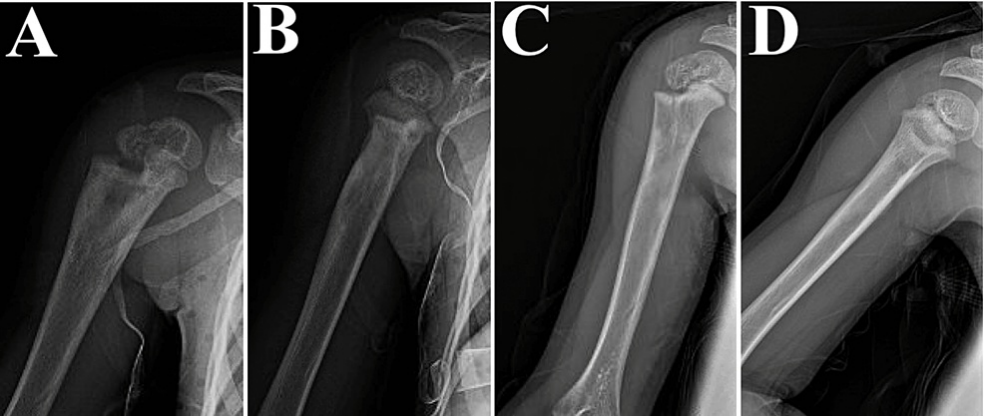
X-rays of the right proximal humerus three months after discharge in AP (A) and lateral (B) views and twelve months after discharge in AP (C) and lateral (D) views AP: Anteroposterior
